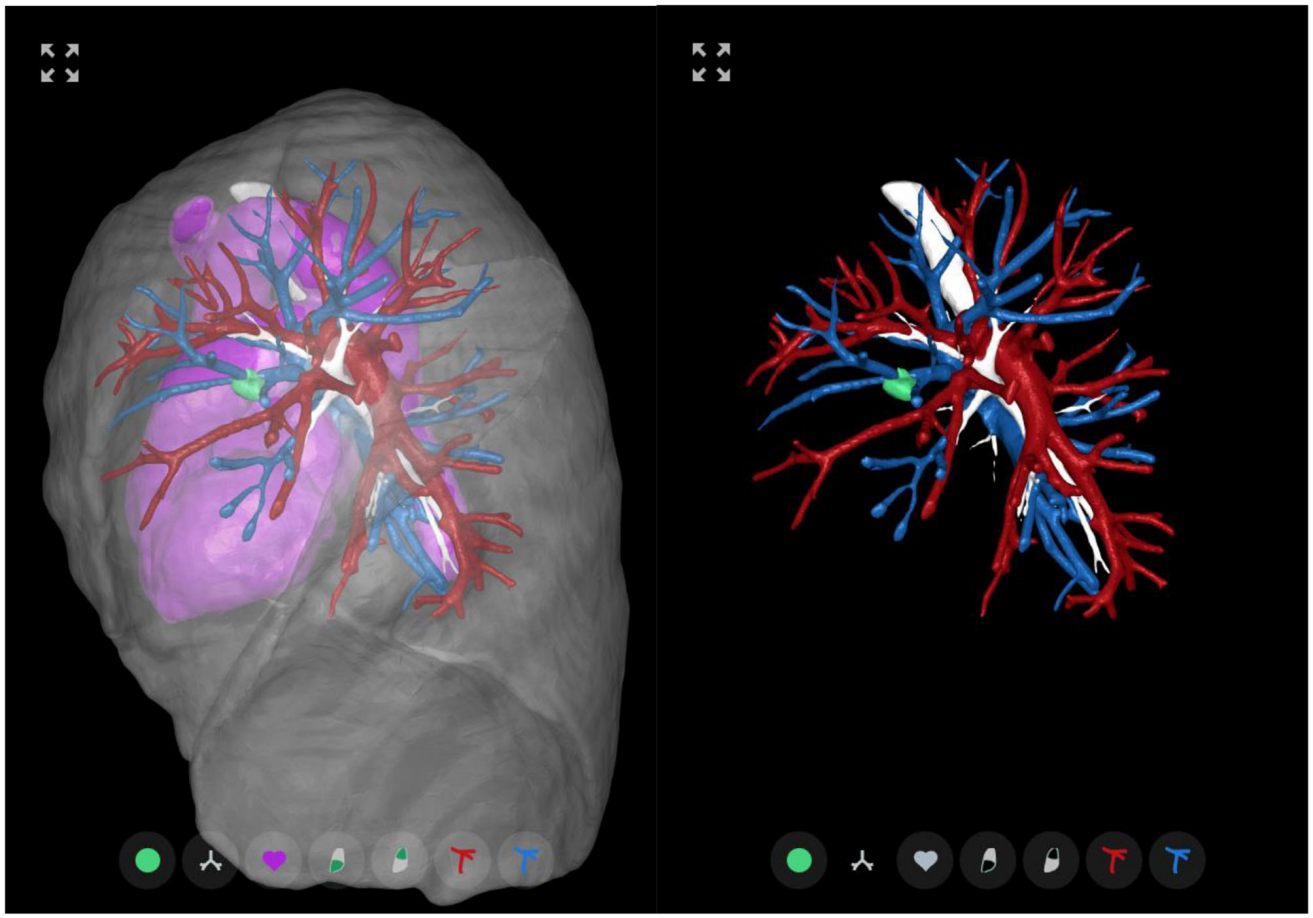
3D-CT example. Sample images representing the 3D-CT model available to the participants for the survey cases. 3D-CT model viewed along the sagittal axis from left-to-right of a patient case with a pulmonary left upper lobe nodule treated by robotic anatomical lung resection. (Left) Default object view with the airways, arteries, veins, heart and nodule shown at full opacity with the lobe surfaces shown in semi-transparency. (Right) To allow for a clearer view of the pulmonary vessels, the heart and lobes have been made fully transparent. In this case, one can see that the lingular artery runs anterior to the bronchus. The structure-to-colour keys are: tumor—green; bronchus—white; heart—pink; lobes—transparent gray; arteries—red; and veins—blue (see online version for color images).
